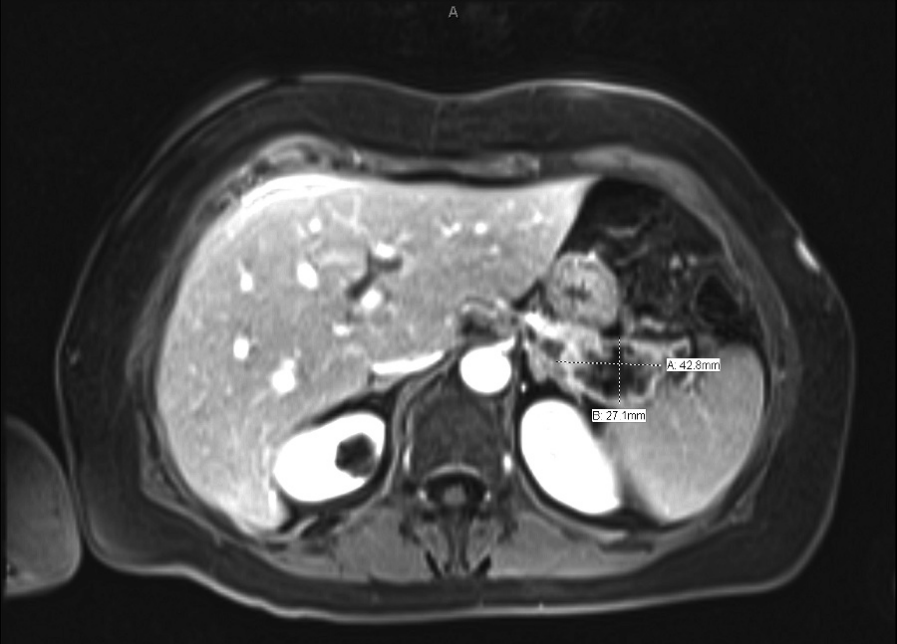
Magnetic resonance imaging demonstrating adenocarcinoma (27.1 mm X 42.8 mm) in the tail of the pancreas
